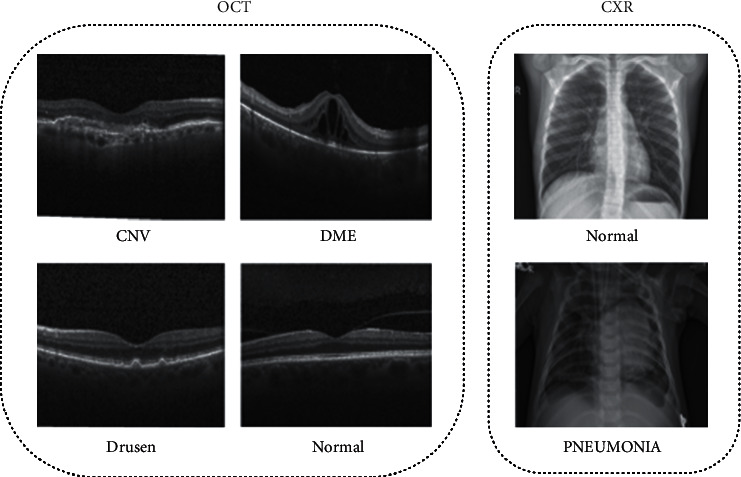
Example medical image samples for classification task from the two selected databases. (a) The optical coherence tomography (OCT) images. (b) The chest X-ray (CXR) images.
